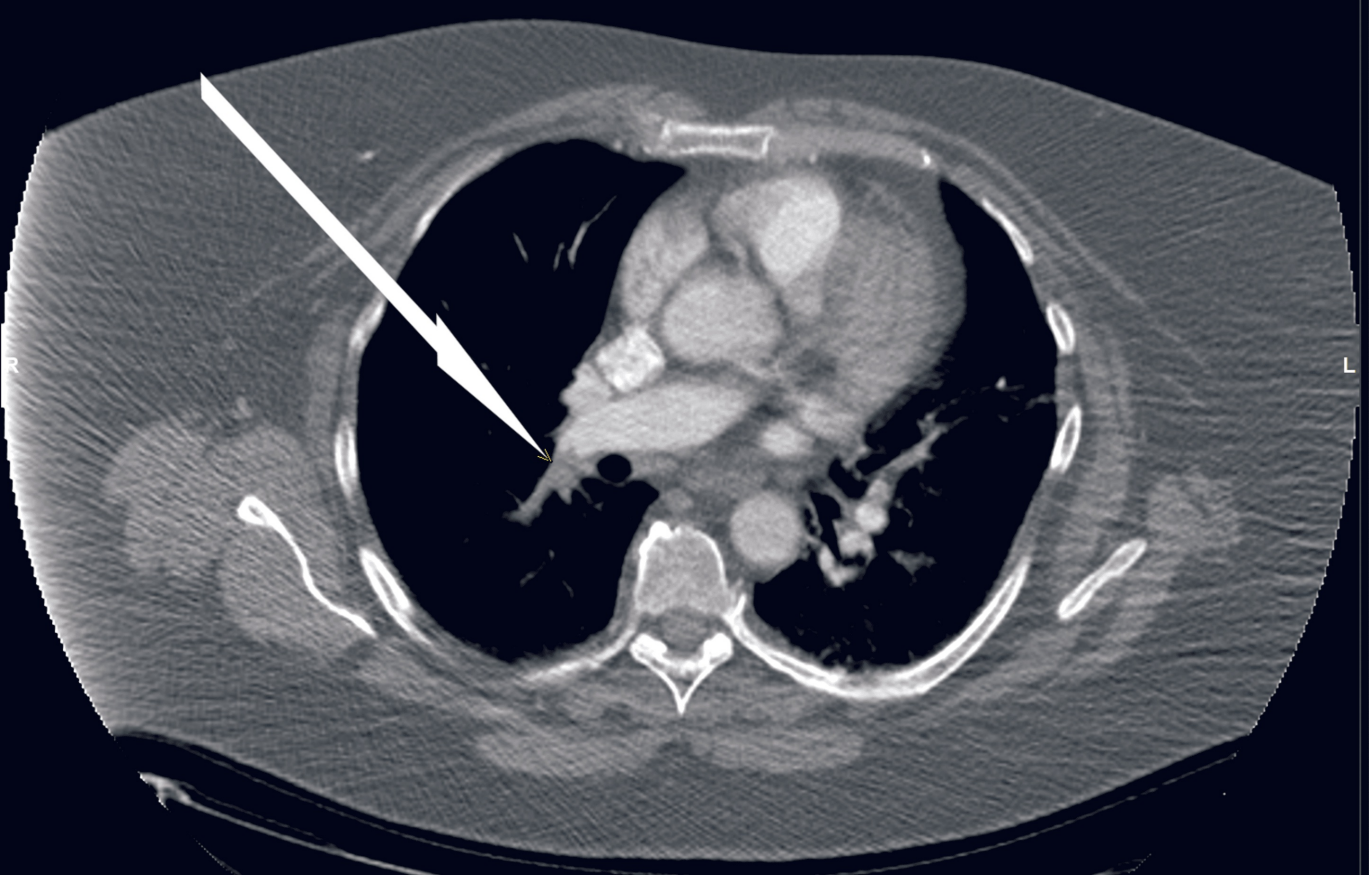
CT pulmonary angiogram, axial view (Case 1). Arrow indicates the grey area without dye in the right pulmonary artery, which is the embolus.
